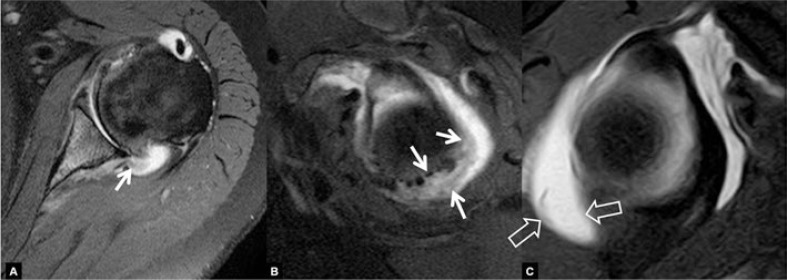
MR arthrographic fat suppressed T1-w MR images in the axial (a) and oblique sagittal (b,c) planes in a patient with a diagnosis of adhesive capsulitis (a,b), there is abnormal tissue in the inferior and posterior axillary recess (arrows) and a limited capacity of the joint which is not distended. In a patient with shoulder instability without any indication of adhesive capsulitis (c), a normal joint space configuration is shown (open arrows).
